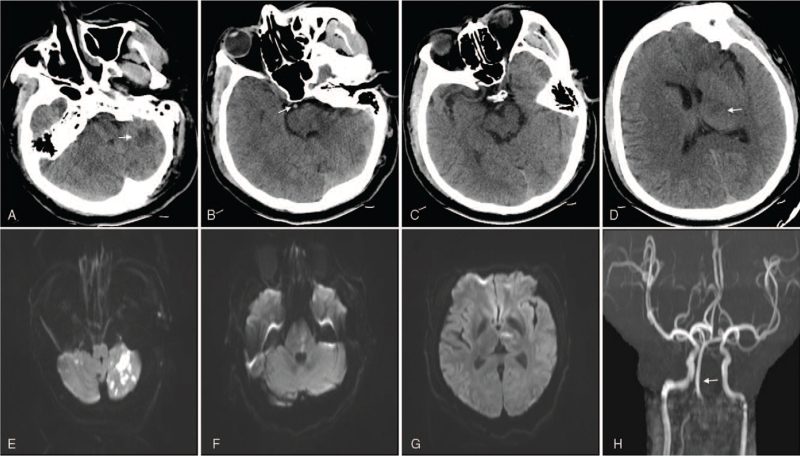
Baseline computed tomography revealed early signs of acute cerebral infarction of the left cerebellar hemisphere (A) and left thalamus (D) (white arrow). Hyperdense basilar artery sign was found (B), but no acute infarction was found in the midbrain (C). Magnetic resonance 24-hour after thrombectomy showed infarcted lesions of the bilateral cerebellum (E), pons (F) and left thalamus (G); blood flow recanalization of the basilar artery (H).
